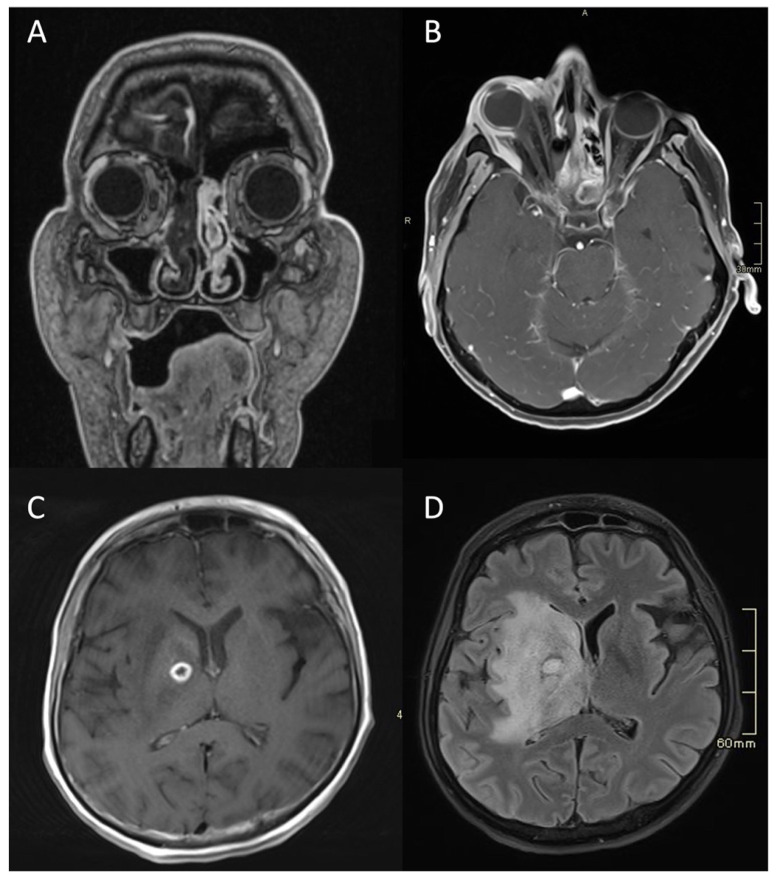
MRI of a patient with an acute fulminant invasive fungal atypical skull-base osteomyelitis due to rhino-orbital-cerebral mucormycosis. (A) First coronal CE MRI T1 showing a lack of a contrast enhancement in the right nasal cavity and paranasal sinuses, indicating necrosis in the context of invasive mucormycotic infection (i.e., black turbinate sign). (B) Axial CE MRI T1 FS two weeks later, showing significant right-sided proptosis, signs of increased intra-orbital pressure, a lack of enhancement in the lateral and partially in the medial rectus muscle, and enhancing and non-enhancing parts of the eye bulb—signs of inflammation and necrosis. (C) Axial CE MRI T1 showing an abscess, which is a ring-enhancing lesion in the brain, with signs of restricted diffusion on DWI (not shown). (D) Axial flair MRI showing extensive oedema and abscess in the deep structures of the right hemisphere three months later. MRI: magnetic resonance imaging; CE: contrast-enhanced; FS: fat-saturation; DWI: diffusion-weighted imaging.
